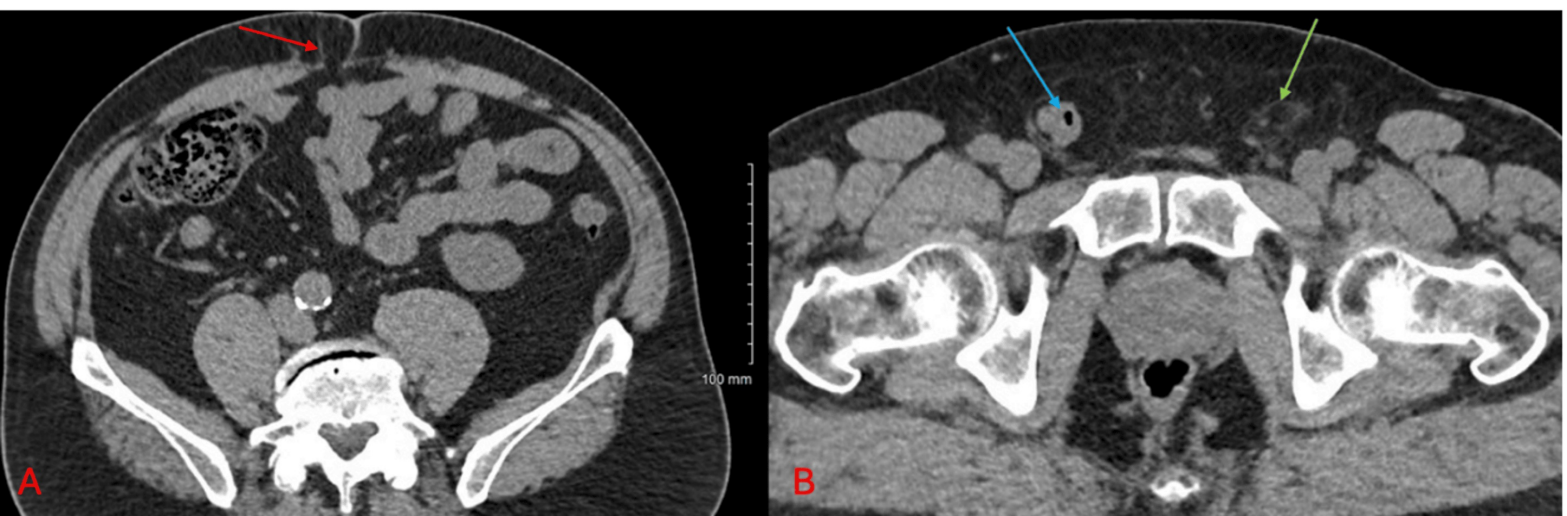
CT of the abdomen and pelvis without contrast (axial view) with a small fat-containing periumbilical hernia in A (red arrow). Panel B shows a small fat-containing left inguinal hernia (green arrow) and a right inguinal hernia containing a loop of the small bowel (blue arrow).
